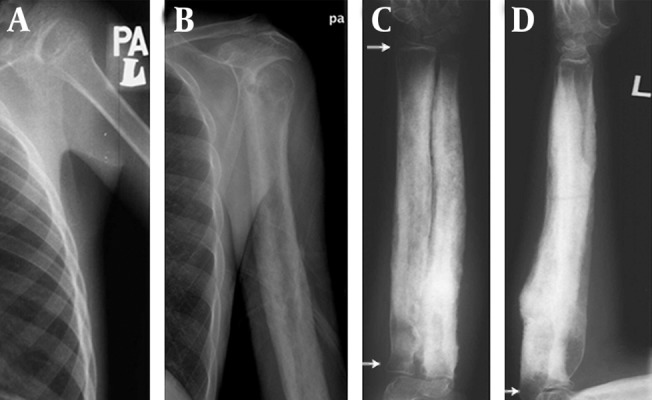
Skeletal Survey A, normal appearance the proximal meta-diaphysis of the humerus had at 15 years of age. B, typical cortical hyperostosis of the humerus meta-diaphysis with sparing of the epiphysis at 28 years of age. C and D, left radioulnar radiograph at the age of 24 years shows meta-diaphyseal hyperostosis of the ulnar and radius with sparing the epiphysis (arrows).
